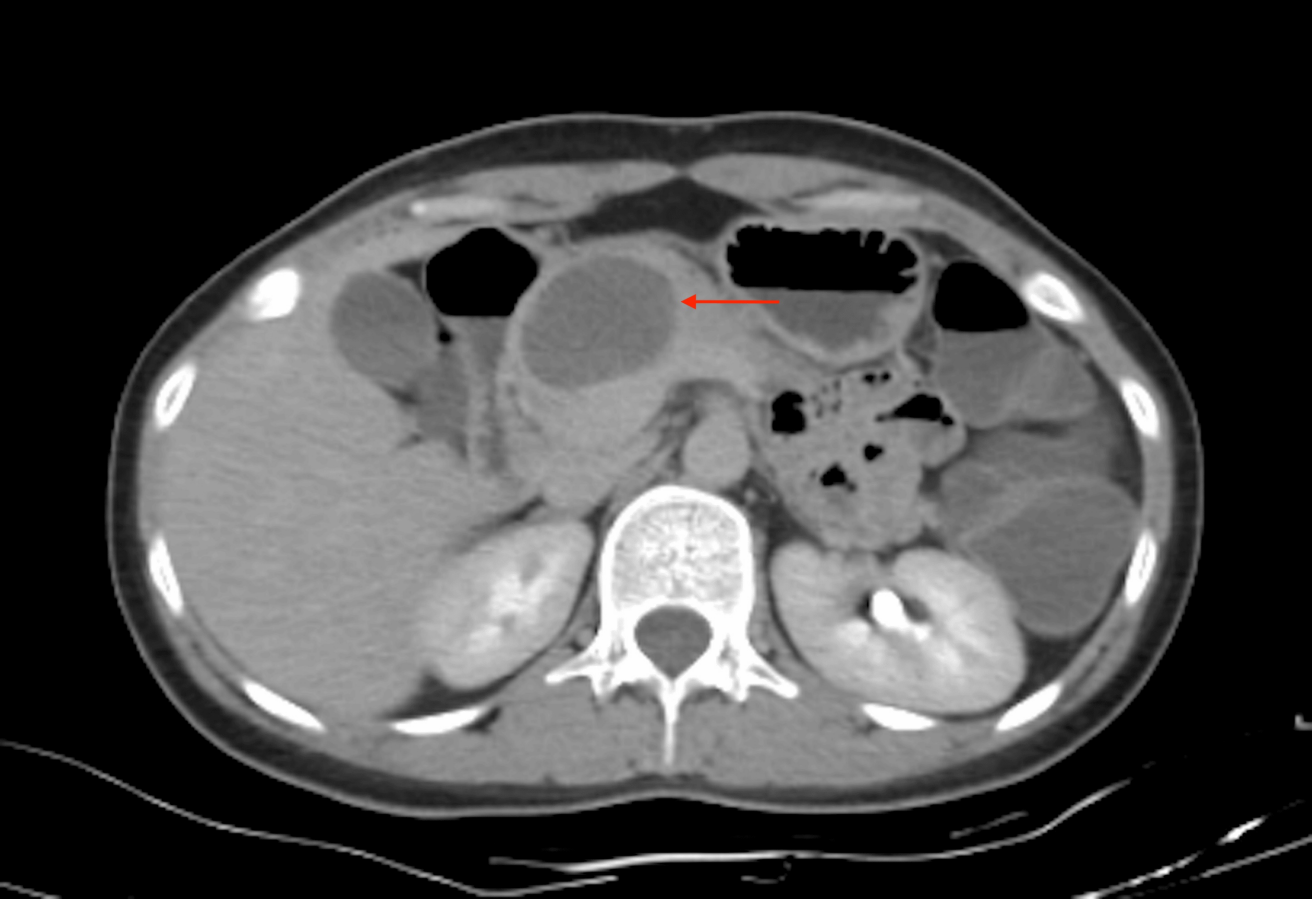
Non-contrast computed tomography (CT) scan of pancreatic mass.
